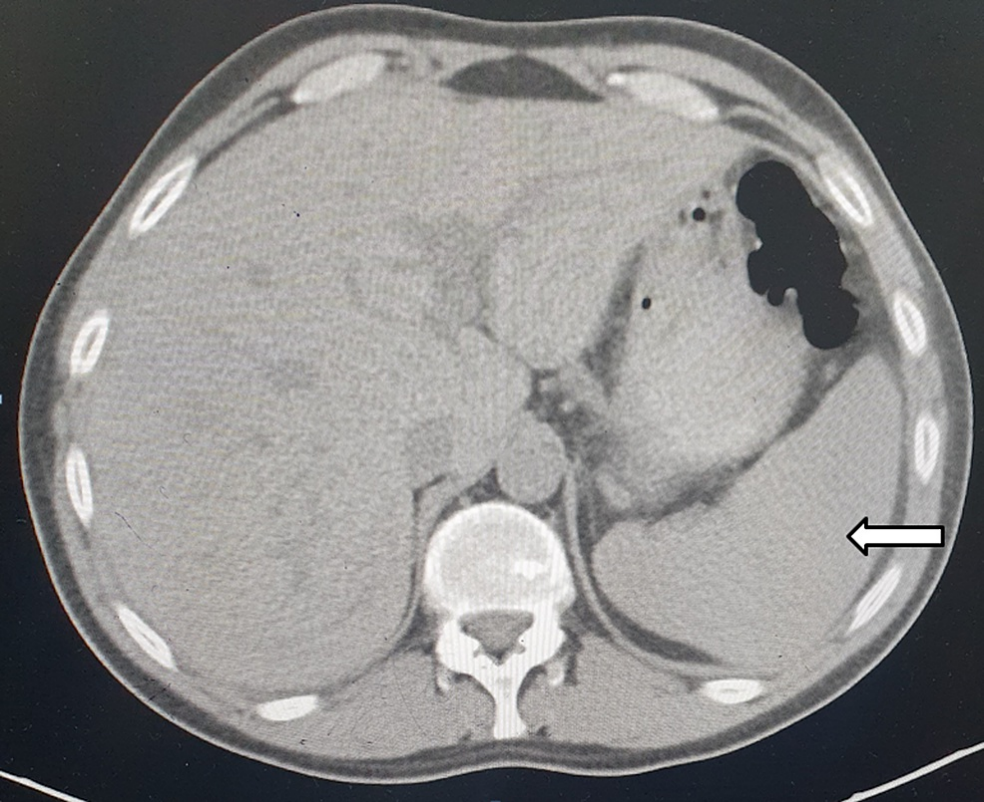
CT scan demonstrating splenomegaly (white arrow)
